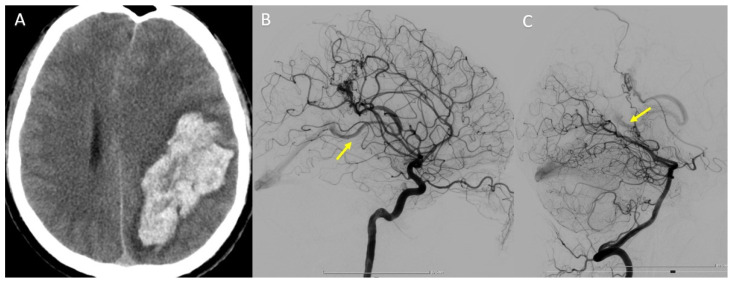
(A) Head CT scan—temporoparietal parenchymal hemorrhage. (B) Left internal carotid (ICA) DSA—lateral view. (C) Left vertebral artery (VA) DSA—lateral view. Yellow arrow—early filling of the deep venous system, and drainage to the great cerebral vein (of Galen).
